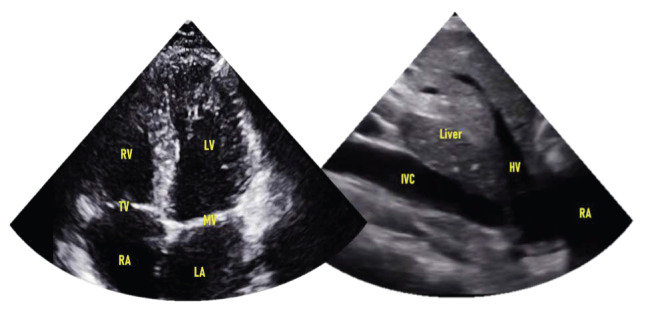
Images of normal cardiac and subcostal views obtainable using a point of care ultrasound (POCUS) examination. The following heart and abdominal structures are labeled: Right and left ventricles (RV, LV), tricuspid and mitral valves (TV, MV), right and left atria (RA, LA), inferior vena cava (IVC), liver,and hepatic vein (HV).
